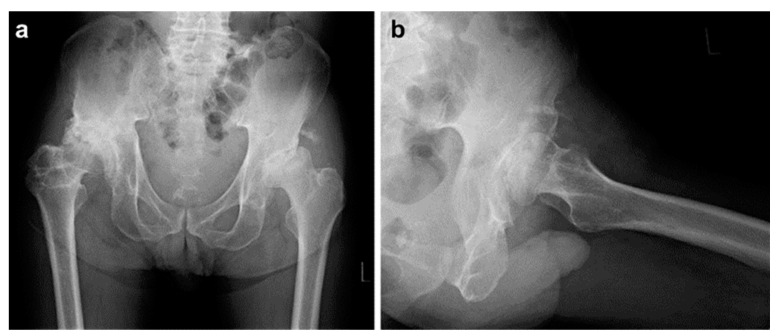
Preoperatively: A 62-year-old male patient was diagnosed with necrosis of the left femoral head. (a) Preoperative anteroposterior plain radiograph of the bilateral hips. (b) Preoperative axial image radiograph of the left hip.
